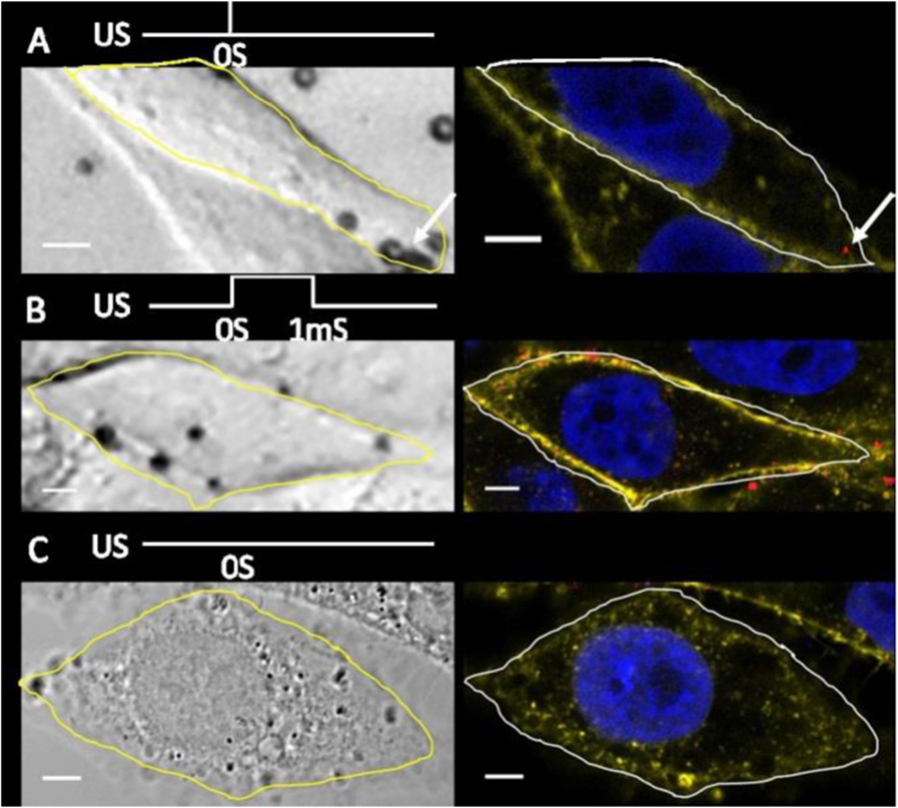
Cy3 labeled plasmid DNA distribution in Hela cells of different ultrasound parameter. Bright field images showing bubble information and representative confocal images showing pDNA distribution with Cy3 labeled pDNA (red), cell nuclei (blue) and membrane(yellow).(A) 0.6MPa,10μs, (B) 0.2MPa,1ms,(C) Control without ultrasound stimulation.Scale bar is 5um
